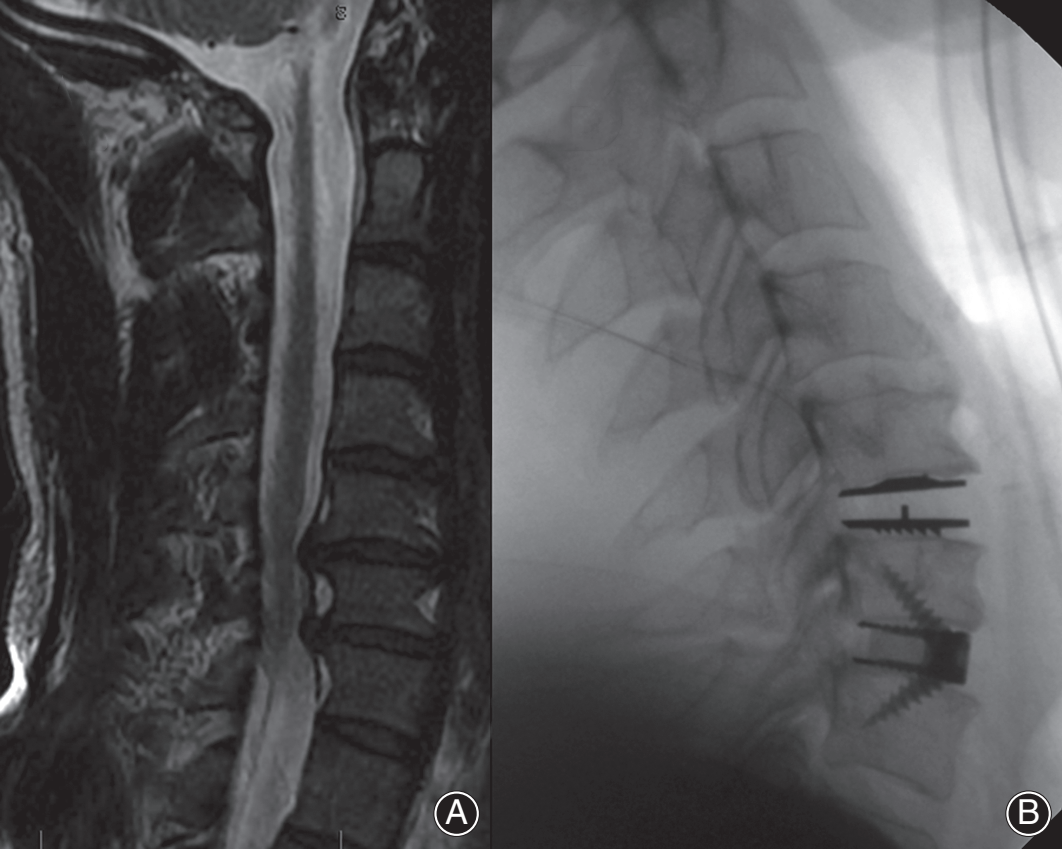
Anterior cervical discectomy and fusion (ACDF)/disc replacement hybrid for disc height loss and foraminal stenosis. (A) Saggital MRI of cervical level. (B) Intraoperative X‐ray.
